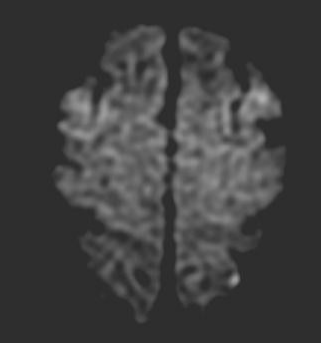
Typical positive DW-MRI (diffusion weighted-MRI) in asymptomatic patient confirming a new lesion appeared after cardiac catheterization.
